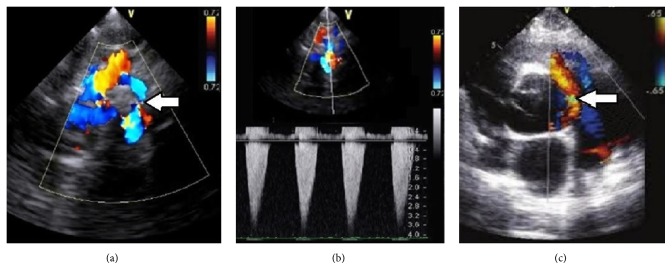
(a) Color flow Doppler imaging echocardiogram on the suprasternal longitudinal view showing coarcted segment of aorta with turbulence (arrow) in the descending aorta. (b) Continuous wave Doppler flow echocardiography demonstrating the coarctation gradient with a peak flow velocity in the descending aorta of 3.8 m/s which corresponds to a maximal gradient of 58 mmHg. (c) The two-dimensional echocardiogram, with color flow Doppler, recorded from the parasternal short-axis view, shows blood flow through a patent ductus arteriosus (arrow).
